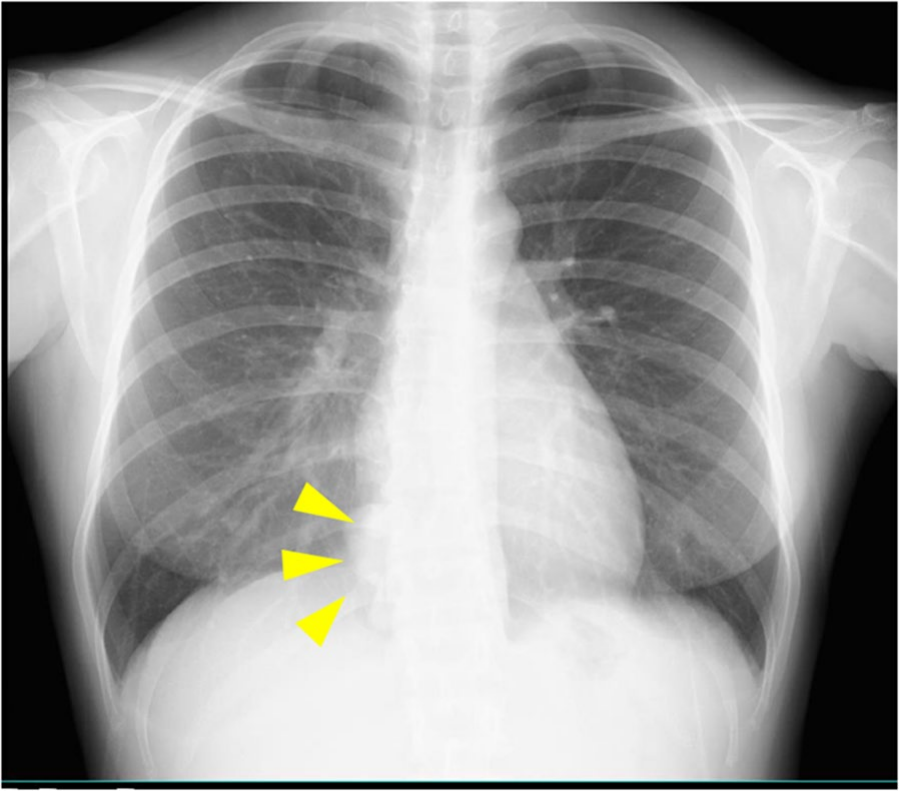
Chest X-ray showing a tumor in the right lower lung field (yellow arrows)
